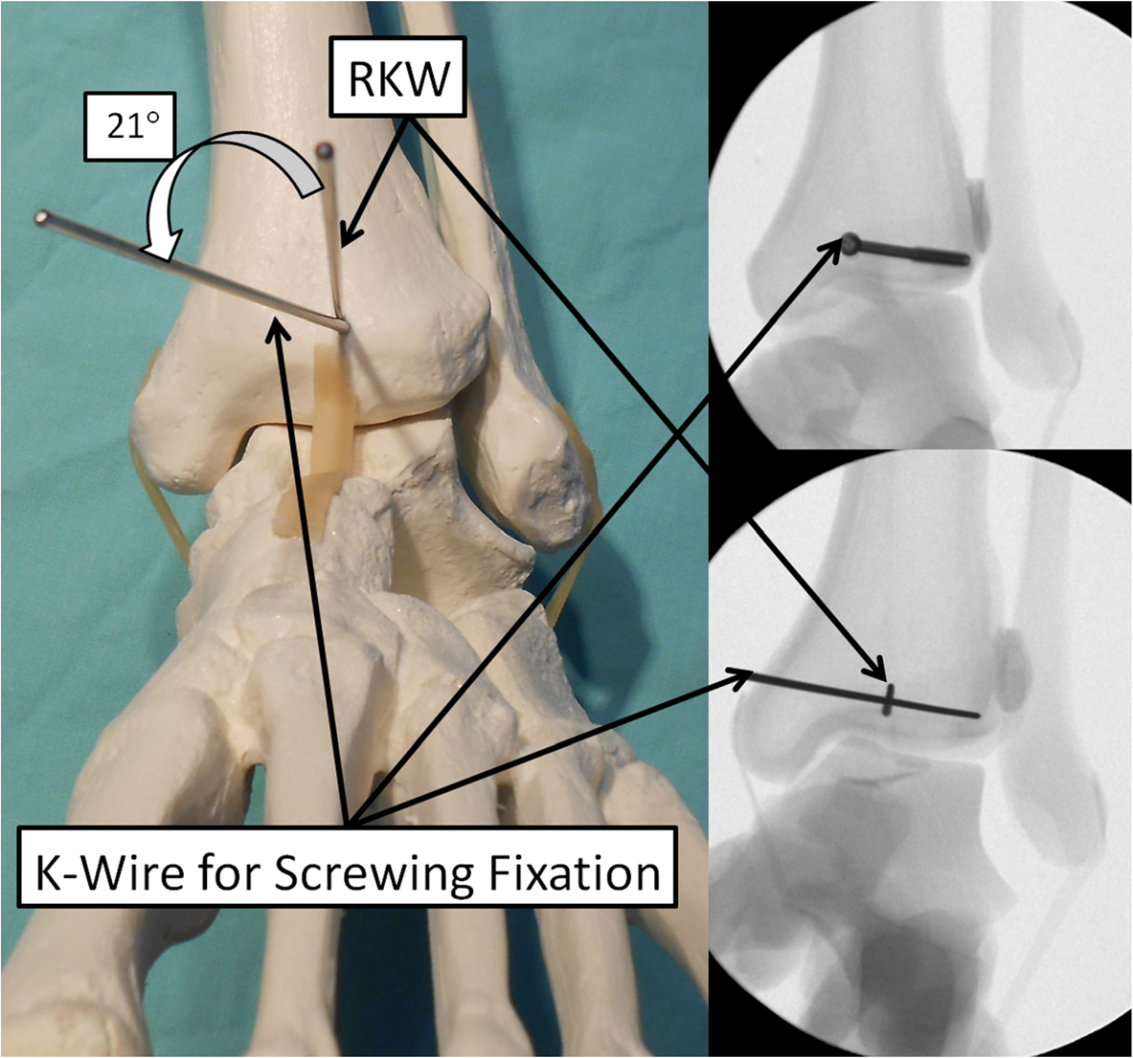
A second K-wire is inserted as a guide-pin for the screw in the correct trajectory angle in order to intersect and adequately stabilize the posterior malleolar fragment. The direction of this K-wire corresponds to the mean trajectory angle of the specific fracture type calculated on the axial CT cut
